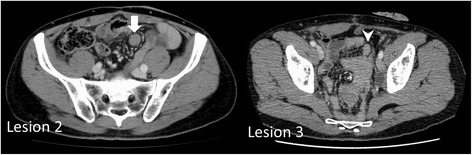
CT findings 3 months after previous CT. Abdominal CT shows two new solitary masses measuring 20 × 15 mm (arrow) and 15 × 10 mm (arrowhead) located at the mesentery of the small bowel
